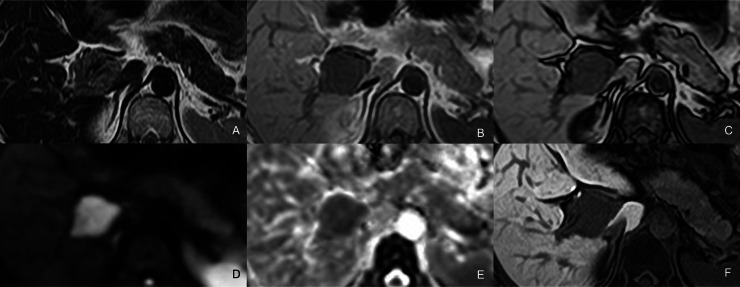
MRI findings. (A) T2-weighted image (T2WI), (B) in-phase and (C) out-of-phase T1-weighted images (T1WI), (D) diffusion-weighted image (DWI), (E) apparent diffusion coefficient (ADC) map, and (F) hepatobiliary phase of gadoxetic acid–enhanced MRI. The mass demonstrates well-defined margins and a fusiform configuration. It appears heterogeneously hypointense on T2WI and homogeneously hypointense on T1WI, with no apparent fat component. The lesion exhibits restricted diffusion. In the hepatobiliary phase (F), the tumor appears hypointense.
